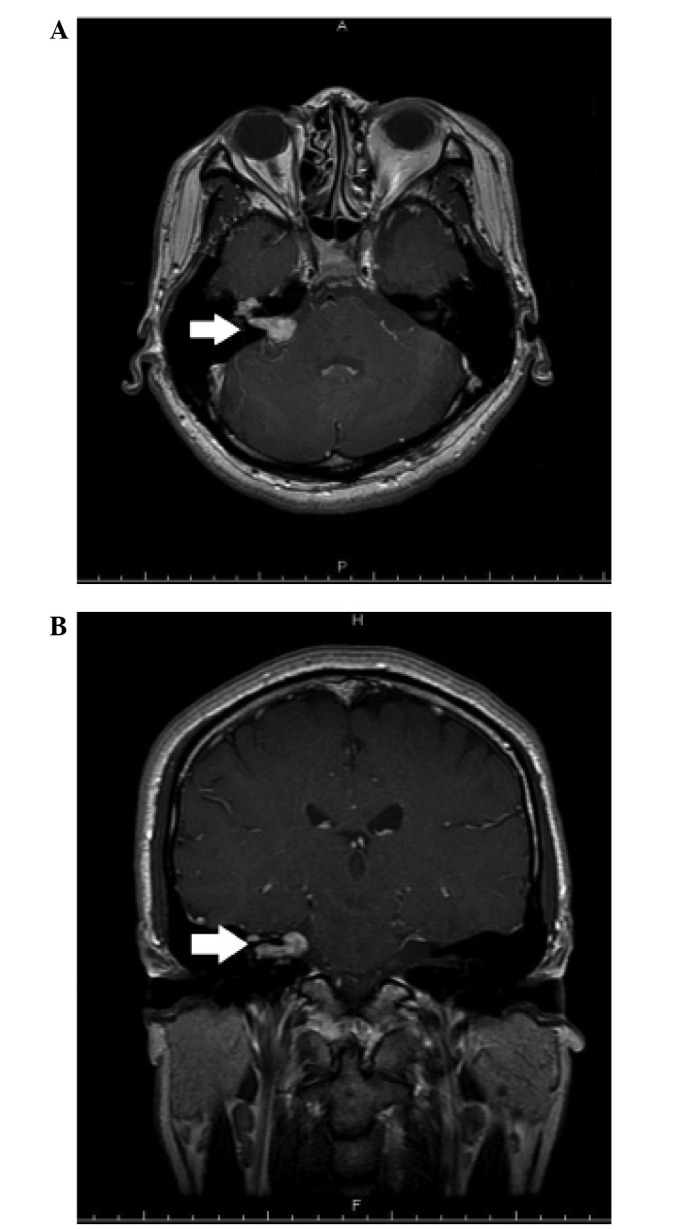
Contrast-enhanced T1-weighted magnetic resonance images. (A) Axial and (B) coronal views demonstrate an enhancing mass at the cerebellopontine angle and internal auditory canal, with involvement of the labyrinthine segment and geniculate ganglion (as shown by the arrows).
